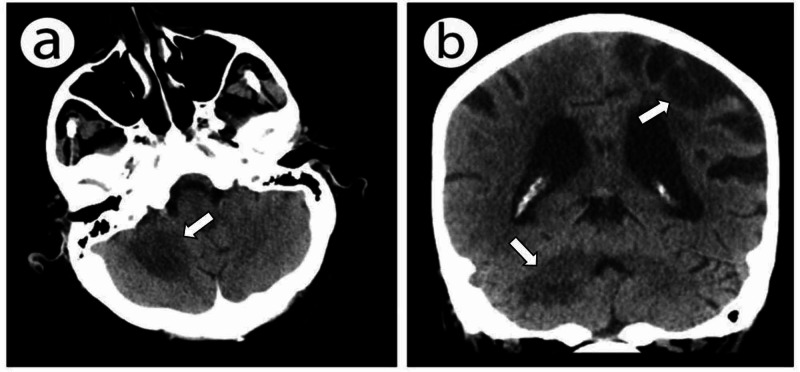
Brain CT axial (a) and coronal (b) sections showing a right cerebellar hypodense lesion with no significant surrounding edema or mass effect and left cortical and subcortical parietal encephalomalacia (on coronal section).
